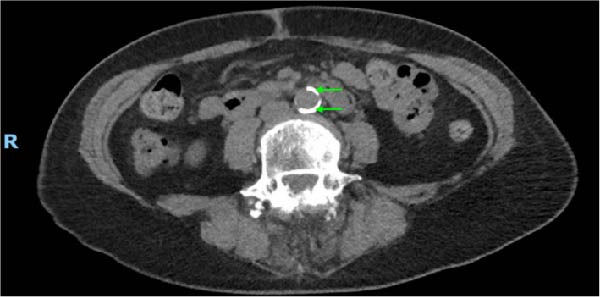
PET/CT F‐18 FDG scan showing mild circumferential low‐grade uptake surrounding the descending aorta.
